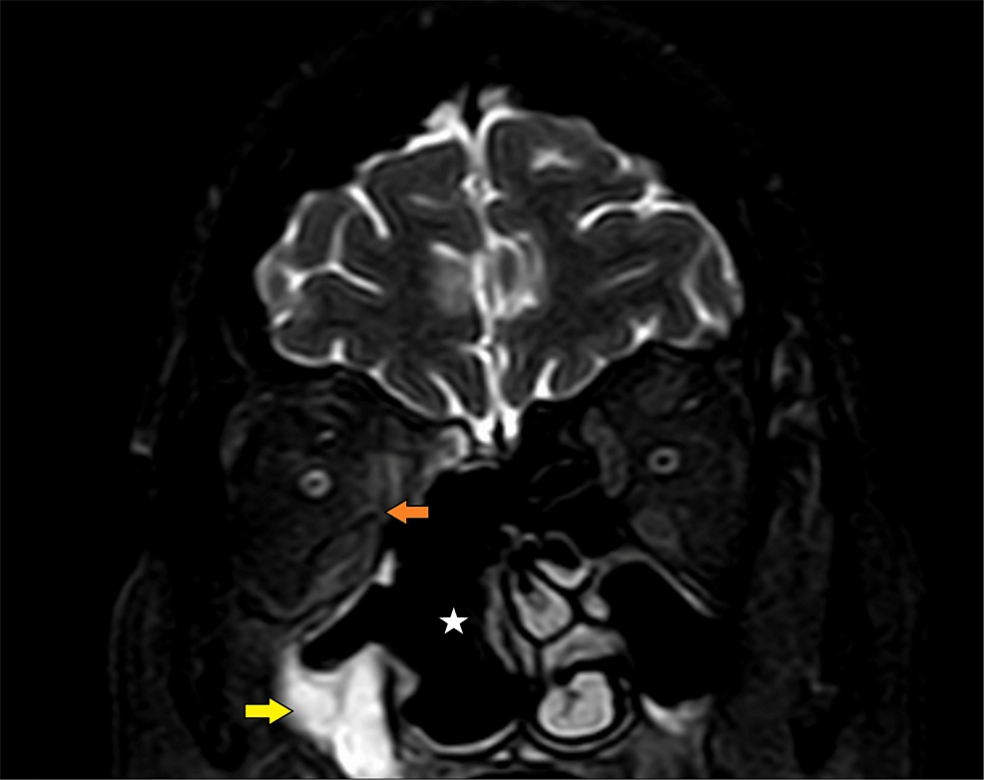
Magnetic resonance imaging (T2 fat-saturated coronal section) showing fluid and mucosal thickening in the right maxillary sinus (yellow arrow), high signals in the inferomedial quadrant of the right orbit (pink arrow), and non-visualization of nasal turbinates on the right side (white star) - postsurgical. Findings are suggestive of the extension of the disease into the orbit.
